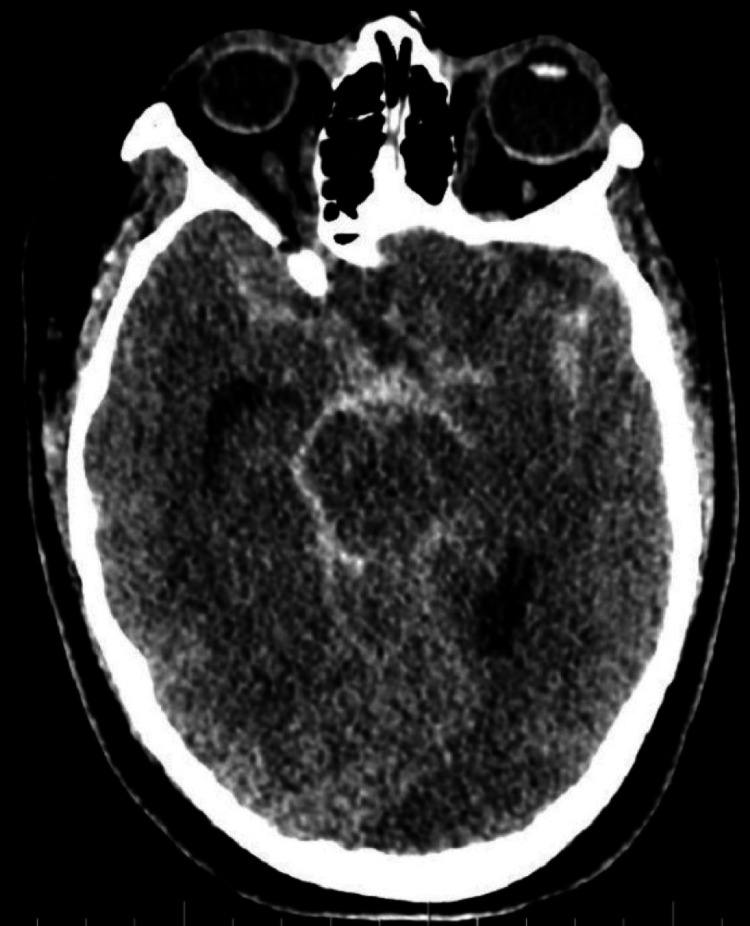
CT brain. CT brain showing subarachnoid hemorrhage. CT: computed tomography.
